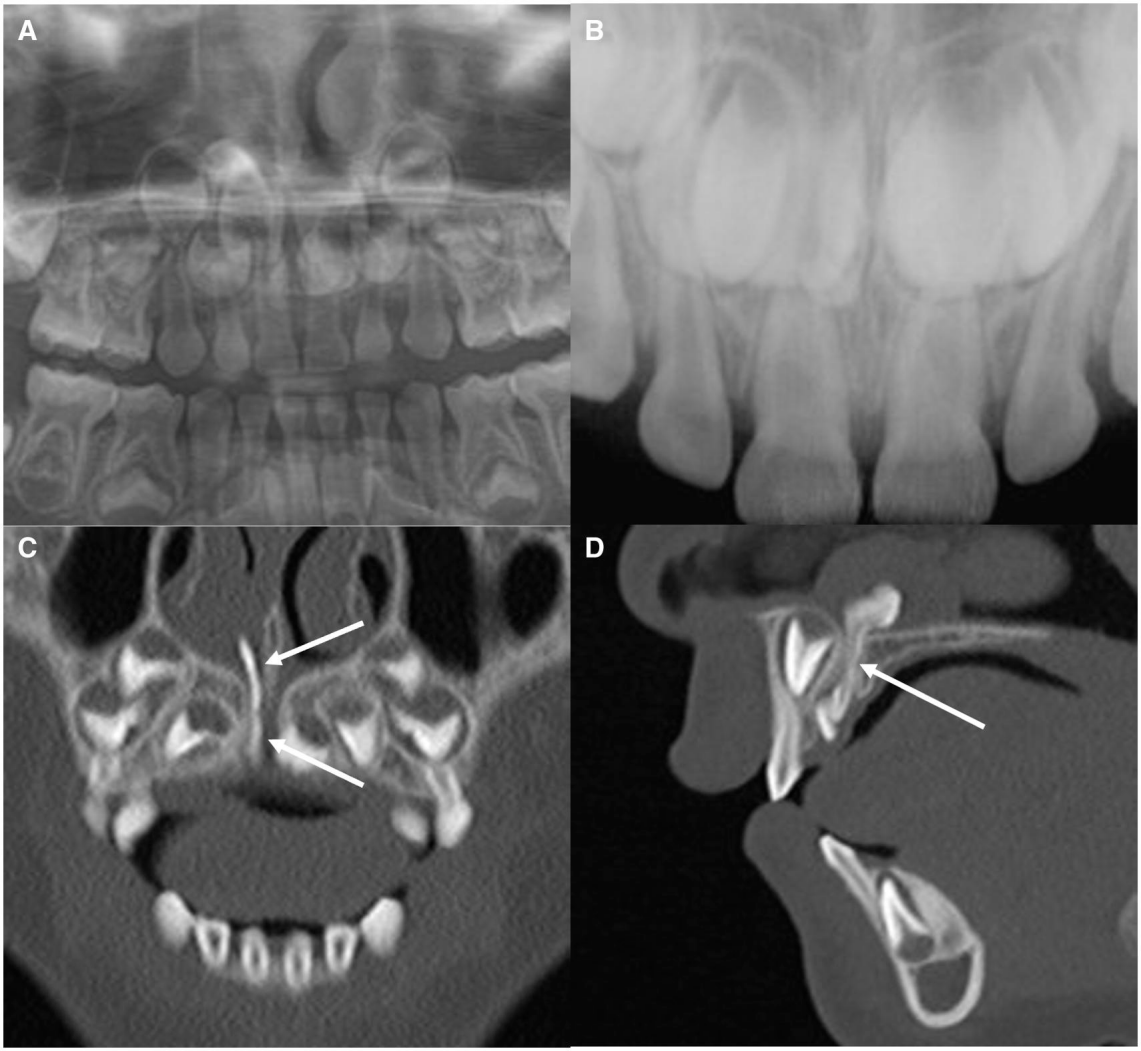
Representative image of fusion in case 14. (A, B) Cropped panoramic and occlusal radiographs, respectively. (C, D) Cropped MDCT images (coronal and sagittal views, respectively). Note the fusion of two crowns of mesiodens within the canal (D, arrow). The presence of mesiodens within the canal and the malformations is not exactly detected on panoramic and occlusal radiographs.
